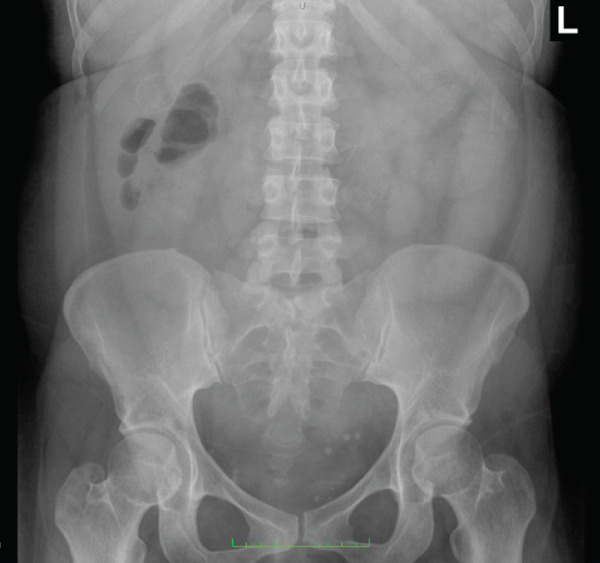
Abdominal radiograph taken on HOD 2 showing no dilated loops of bowel, indicating no clear evidence of an obstructive process at this time.
